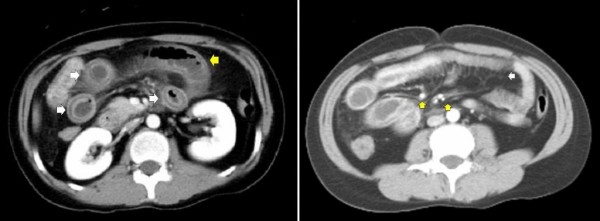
Computed tomography scans of the abdomen. (A) Left image shows distension of the bowel (yellow arrow), diffuse bowel wall thickening (maximum of 8.4 mm), abnormal bowel wall enhancement (double halo or target sign, white arrow). (B) Right image shows mesenteric edema, engorged mesenteric vessels (yellow arrow), prominence of mesenteric vessels with a palisade or comb-like arrangement (comb sign, white arrow). Pneumatosis cystoides intestinalis was absent.
